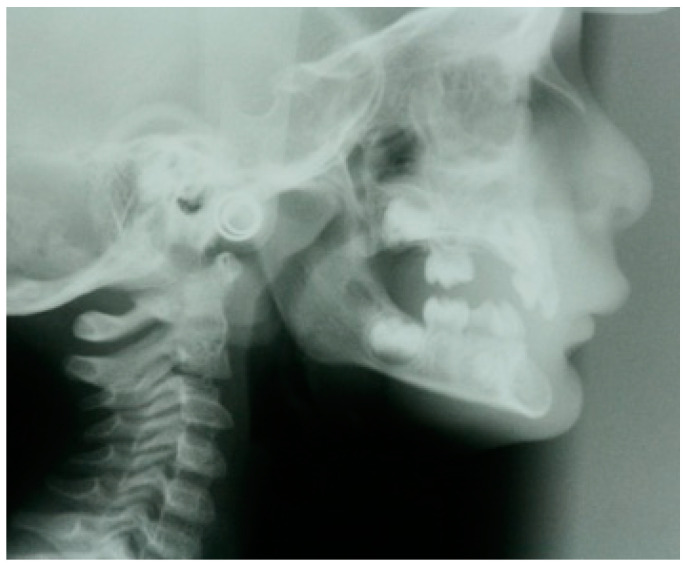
X-ray in a child with oligodontia. Note the characteristic chin projection.
